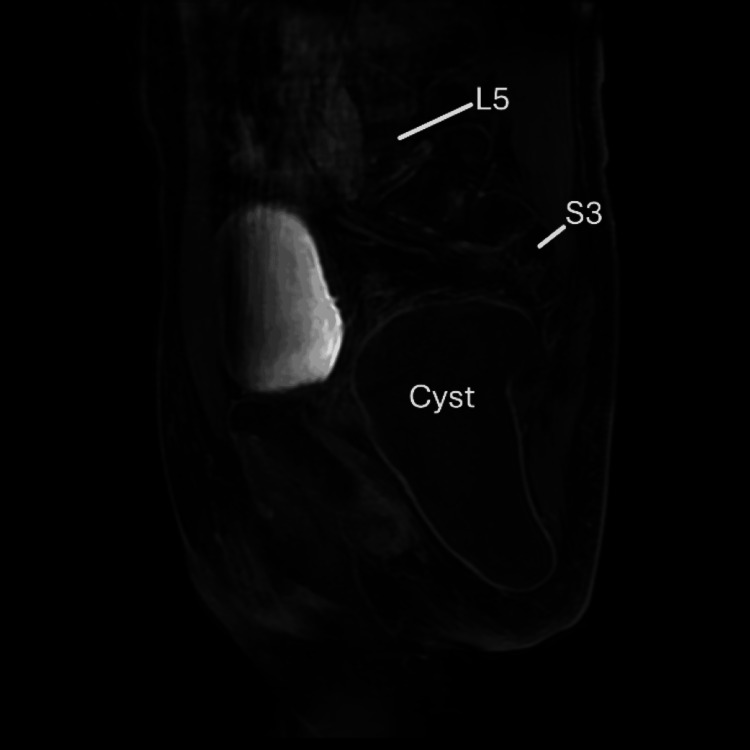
Sagittal cut of MRI MRI: magnetic resonance imaging
